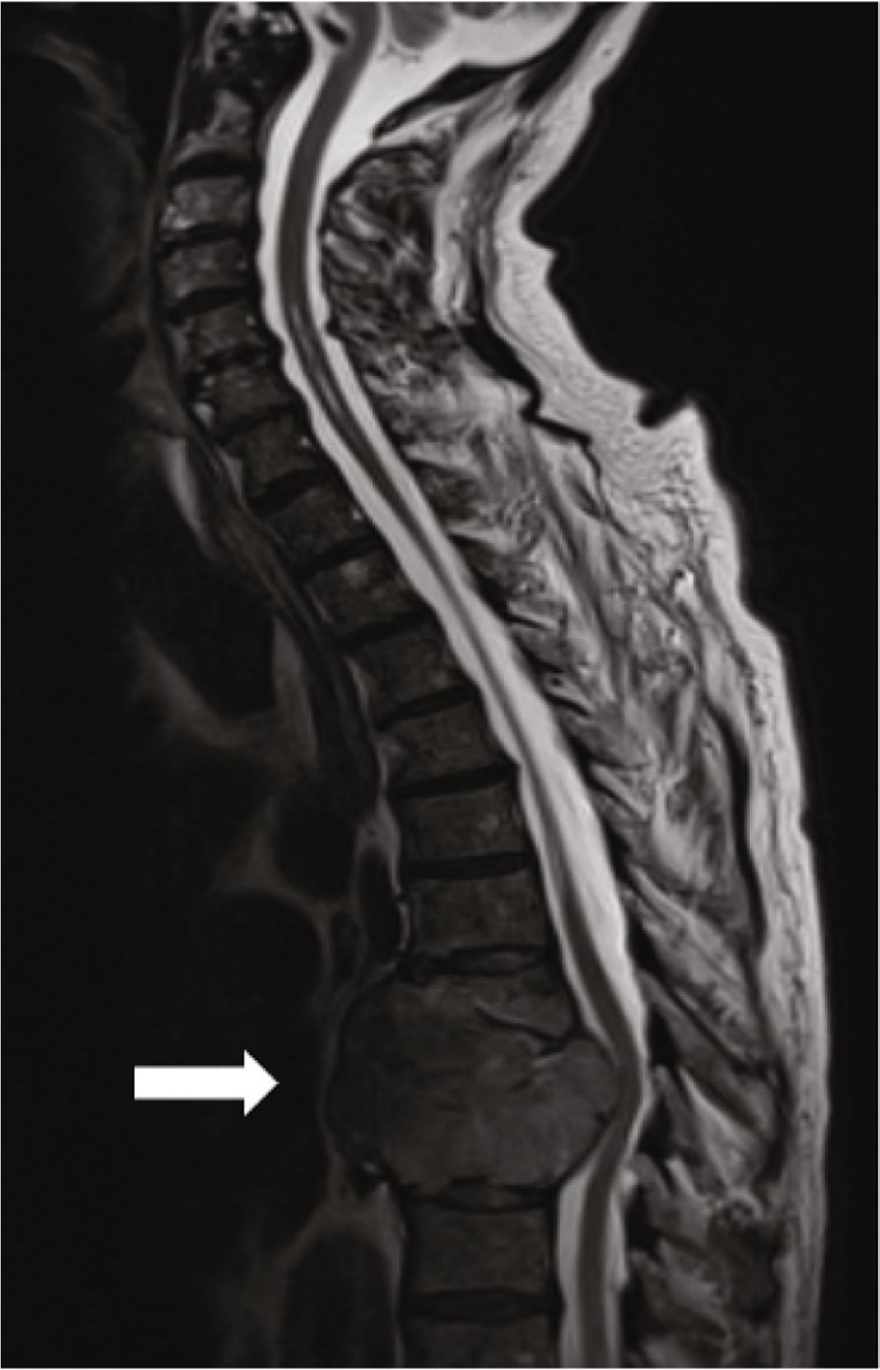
Spinal magnetic resonance image show plasmacytoma (white arrow) encapsulating the whole of Th8 with severe spinal cord compression with subsequent myelopathy
